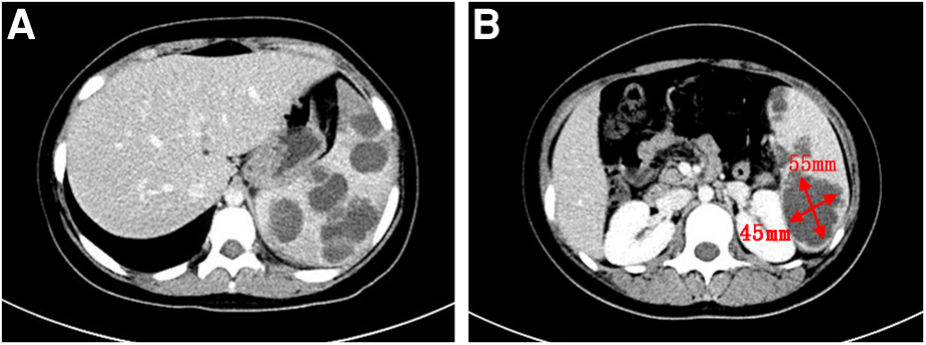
Contrast-enhanced abdominal CT scan. (A): Multiple low-density lesions of the spleen. (B): The largest lesion measures 45 × 55 mm.
